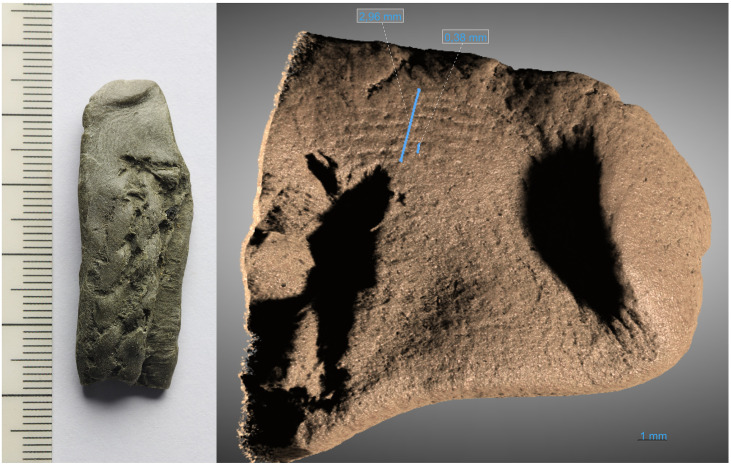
Photo of caulking fragment showing fingerprint on the left and high-resolution x-ray tomography scan of fingerprint region on the right. Photography by Erik Johansson, 3D model by Sahel Ganji.
